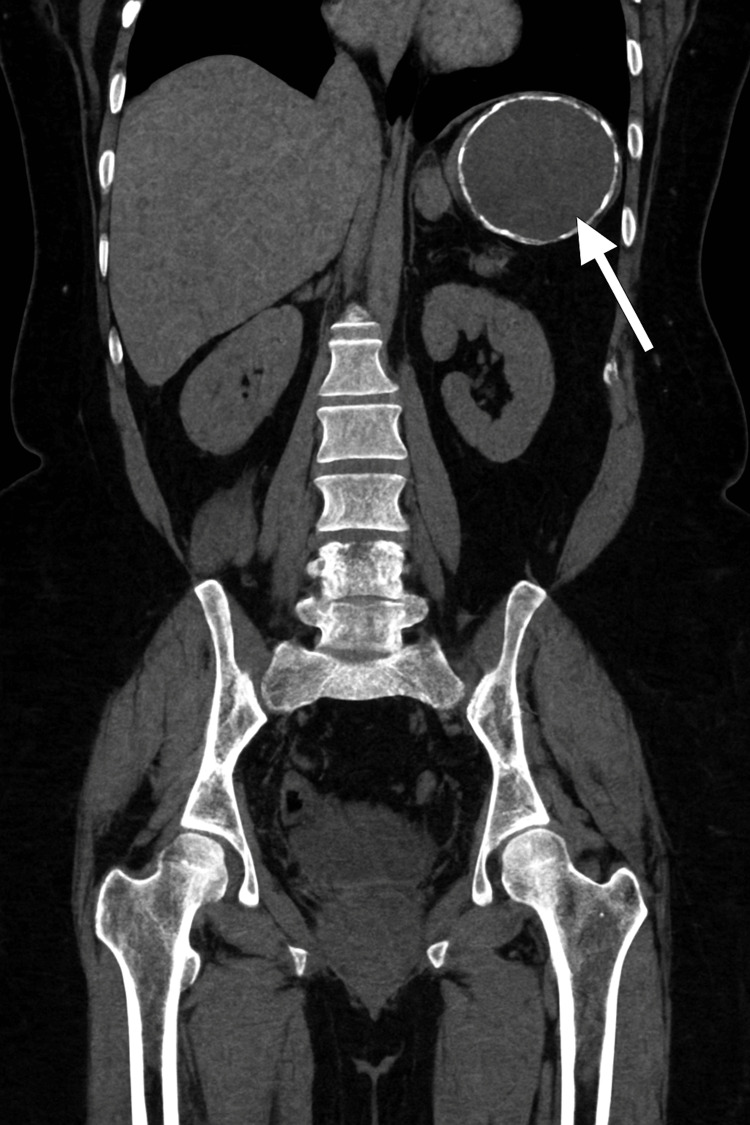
Coronal contrast-enhanced CT scan demonstrating a splenic epithelial cyst A well-defined, unilocular cystic lesion is seen in the upper pole of the spleen (white arrow), measuring approximately 6–7 cm, with no internal septations or solid components. Findings are consistent with a benign epithelial cyst.
